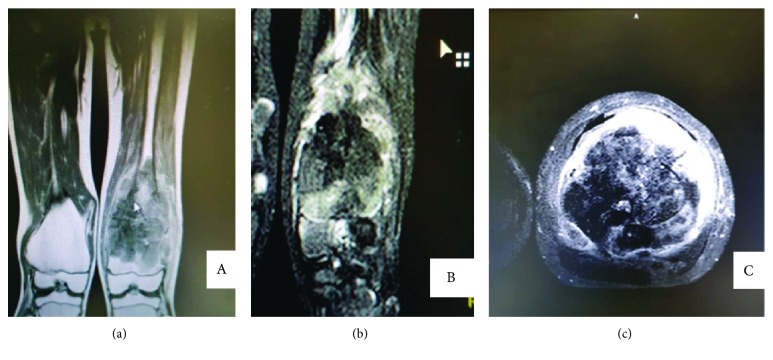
MRI with (a) coronal T2W1 demonstrates heterogeneous signal intensity, (b) coronal T1W1 with contrast, and (c) axial T1W1 with contrast demonstrates moderate heterogeneous enhancement. There were no skip lesions.
